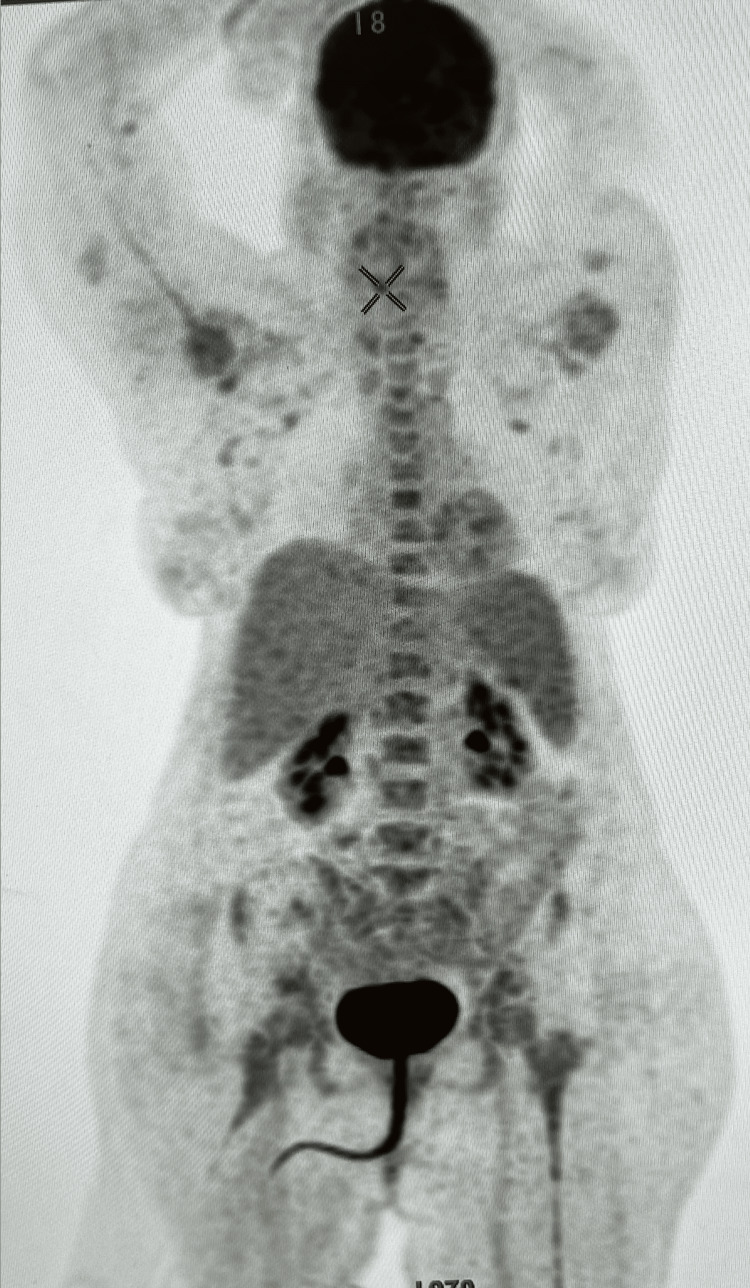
A thorough nuclear medicine PET/CT scan unveils moderate uptake patterns in the bone marrow and spleen, potentially signifying an underlying autoimmune state, nearly excluding underlying malignancy, all-encompassing from vertex to mid-thigh PET: positron emission tomography
